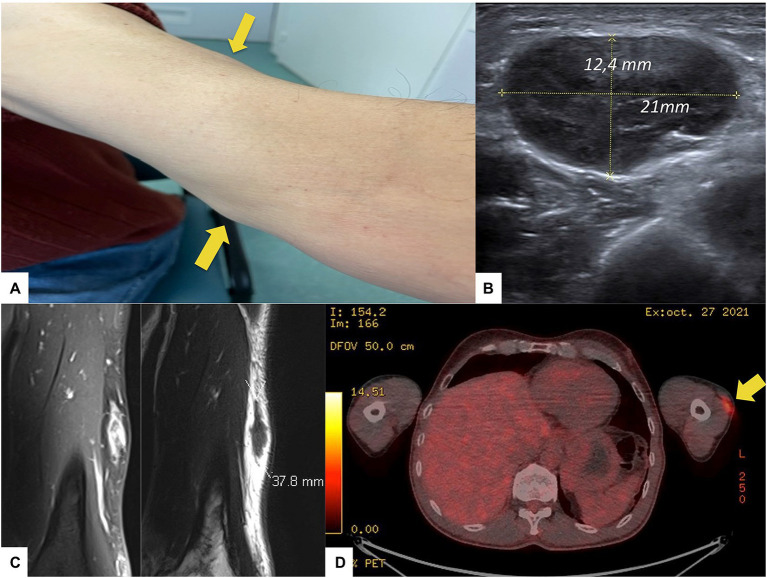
Clinical and paraclinical features of the patient. (A) Presence of two subcutaneous nodules on the left arm (arrows), (B) Soft tissue ultrasound of the left arm evidencing and measuring one subcutaneous mass, (C) MRI frontal slices of the left upper limb (T1 with gadolinium injection on the left, and T2 on the right), and (D) 18-FDG-PET-CT scan showing a 18-FDG uptake by the nodule (arrow) on the left arm.
